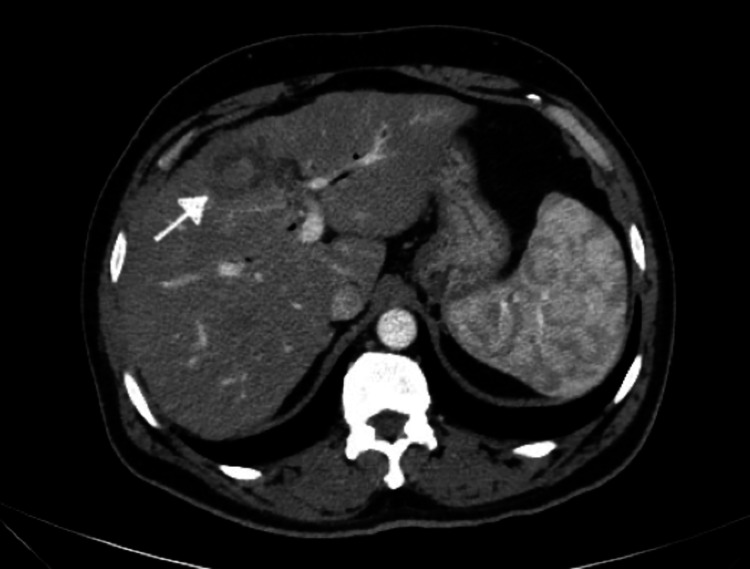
CT abdomen done prior to starting second-line gemcitabine/nab-paclitaxel (Case 1) The arrow indicates liver metastasis.
